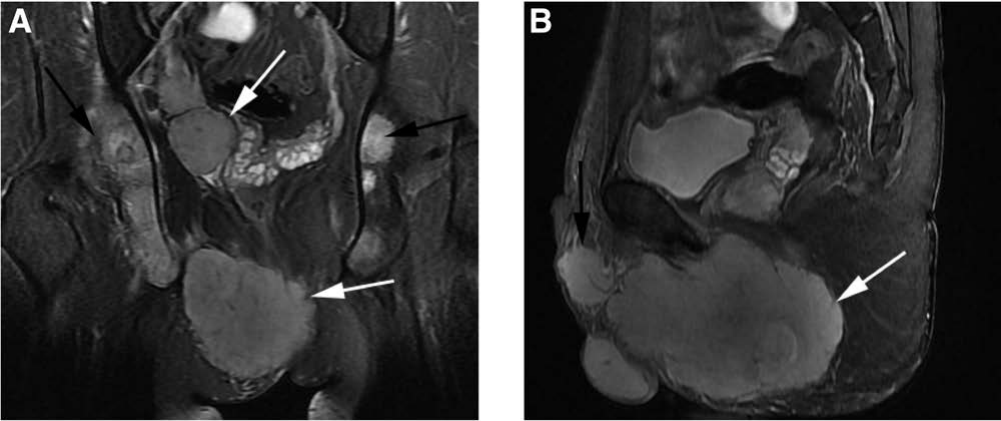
The tumor mass and metastatic sites in MRI T2WI. The tumor mass in pelvic cavity (white arrow) and metastasis in bone (black arrow) in coronal plane (A). The tumor mass in pelvic cavity (white arrow) and metastasis in right testis (black arrow) in sagittal plane (B). MRI = magnetic resonance imaging, T2WI = T2 -weighted imaging.
